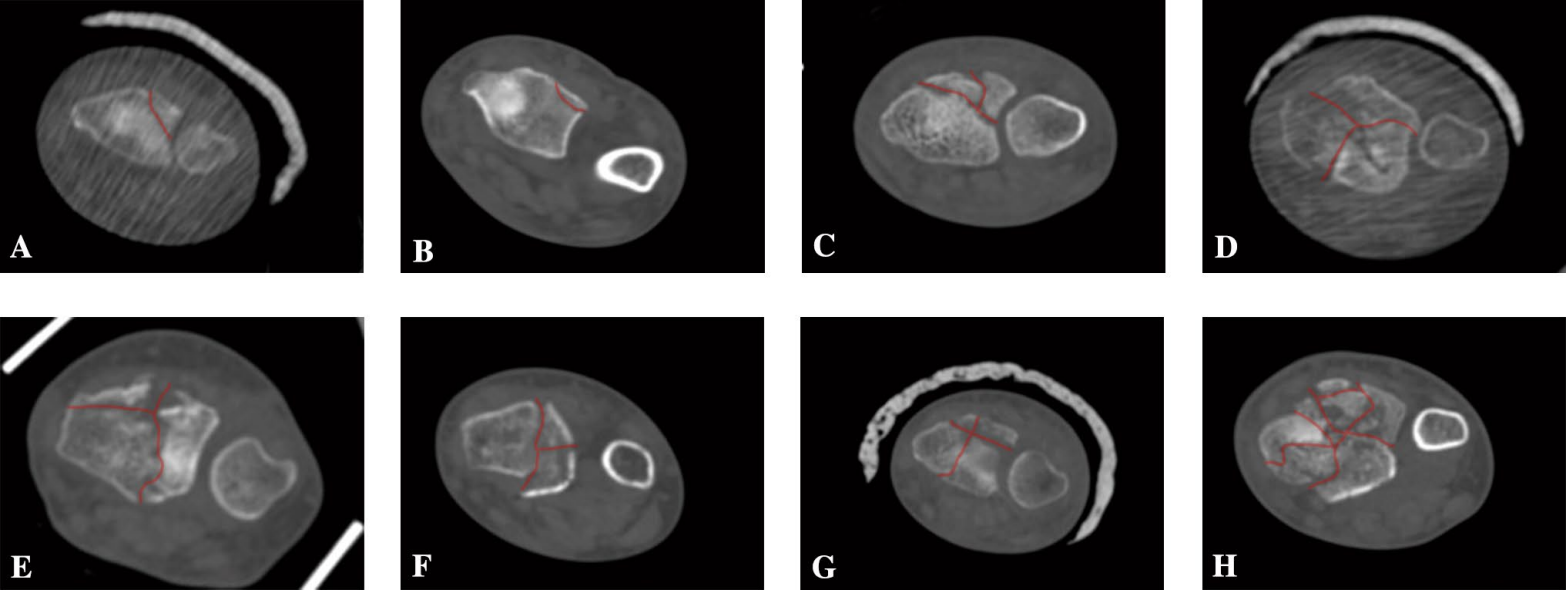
Showing the typical manifestations of our classification of distal radius intra-articular fractures in CT images. The fracture line marked with red line. a, b the fracture of a single fracture line; (C) a typical “┴” type of fracture; d a typical “┬” type of fracture; e a typical “┤” type of fracture; f a typical “├” type of fracture; g a typical “┼” type of fracture; h the fracture of multiple fracture lines
